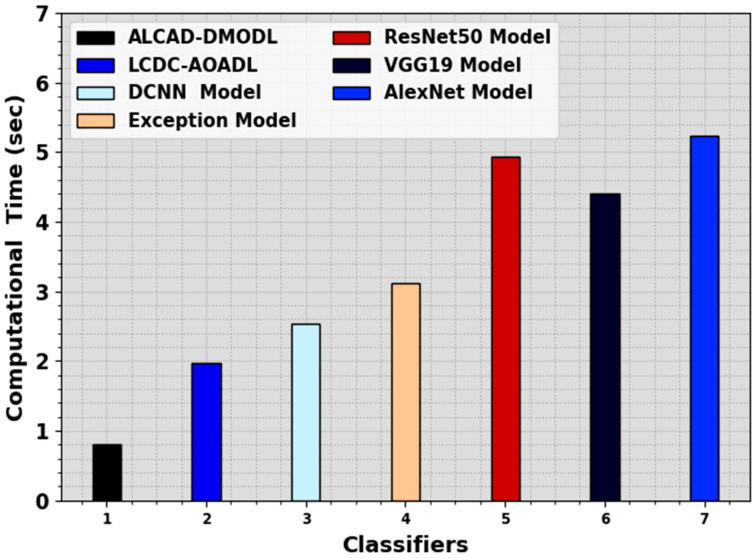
CT analysis of the ALCAD-DMODL system with other models.
